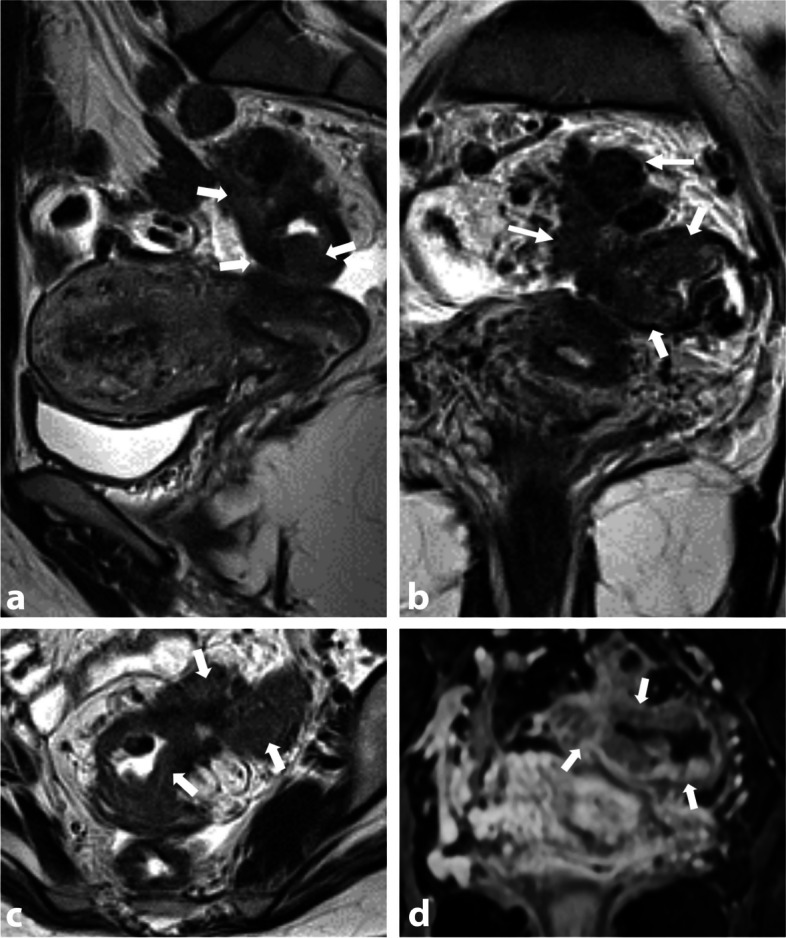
Rectal adenocarcinoma in a 33-year-old patient with chronic pelvic pain and rectal disorder with rectal bleeding, addressed for suspicion of endometriosis. a Sagittal, (b) coronal, and (c) axial T2-W MR images show a T2-hypointense focal wall thickening of the high rectum (thick arrows) with a T2-hypointense tumoral infiltration of the mesorectum (thin arrows). Note the absence of retrocervical deep infiltrating endometriosis. d Axial T1-W fat-suppressed contrast-enhanced MR image shows a moderately enhanced tumor (arrows)
